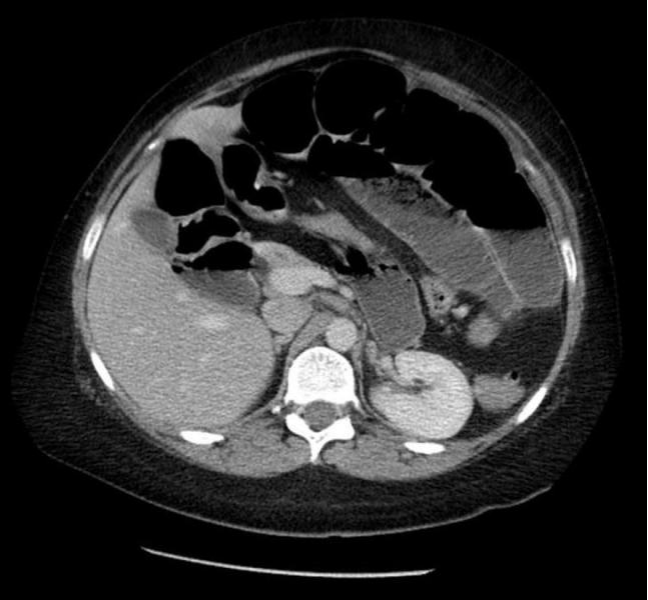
This is a CT Scan showing malrotated large loopy colon and distended small bowel, taken one day before operation performed on the patient.
